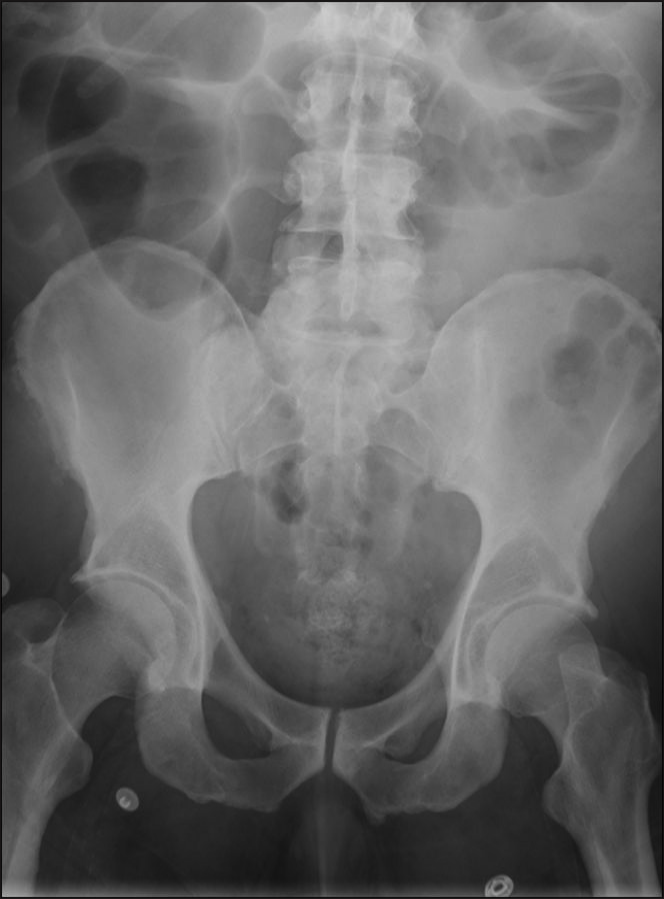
Follow-up radiograph of abdomen showing complete disappearance of air following treatment
